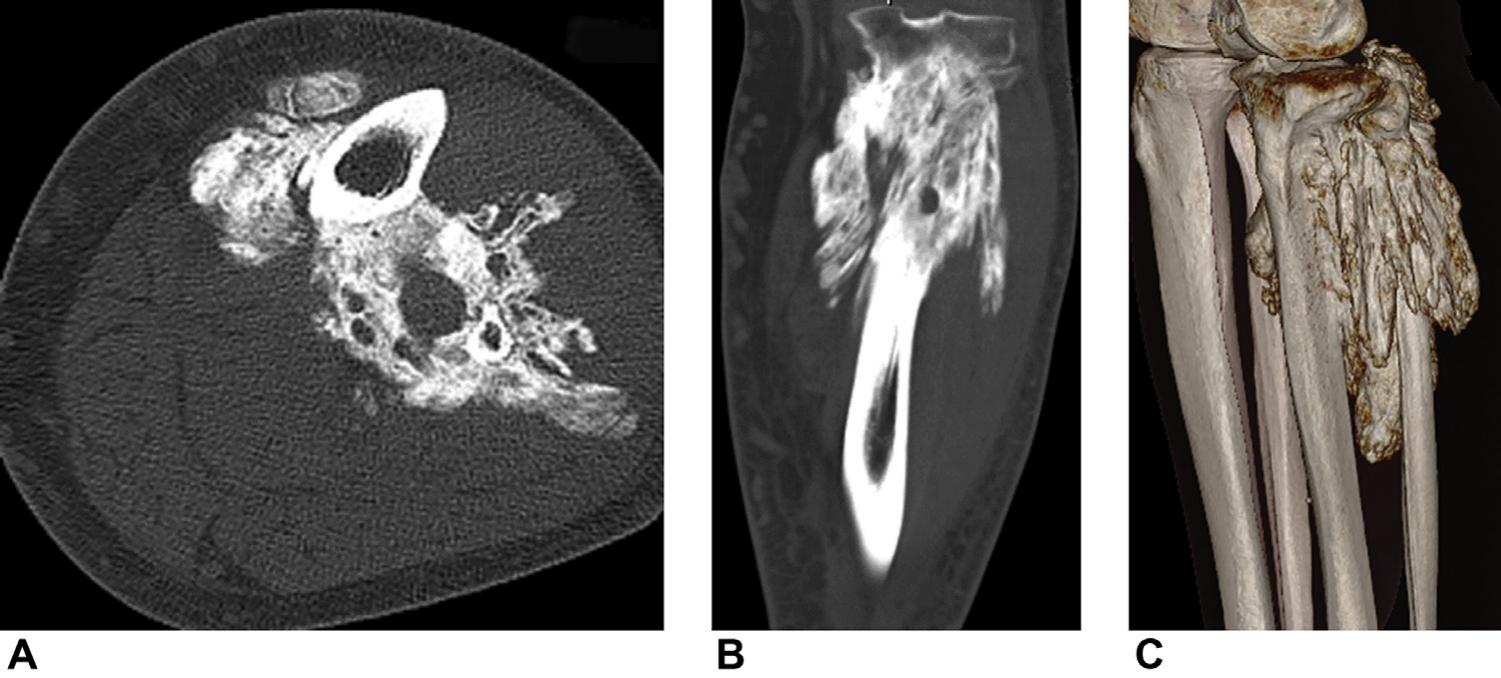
Initial CT examination (A) axial, (B) coronal, and (C) VRT image showing a well-defined, lobulated, mixed density lesion (ossified with several radiolucent soft-tissue areas) attached to the proximal part of the tibia and encircling fibula and popliteal artery and vein.
